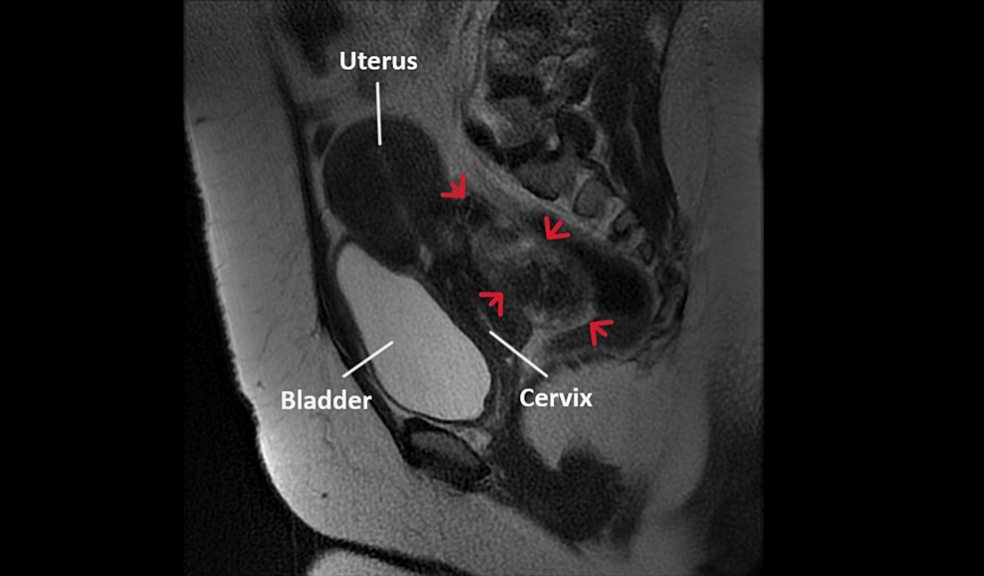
MRI of a posterior pedunculated extracervical leiomyoma of the uterus The pelvic space-occupying lesion depicted with red arrows represents an extracervical-type posterior pedunculated leiomyoma of the uterus, whose extension into the retroperitoneal space cannot be clarified
